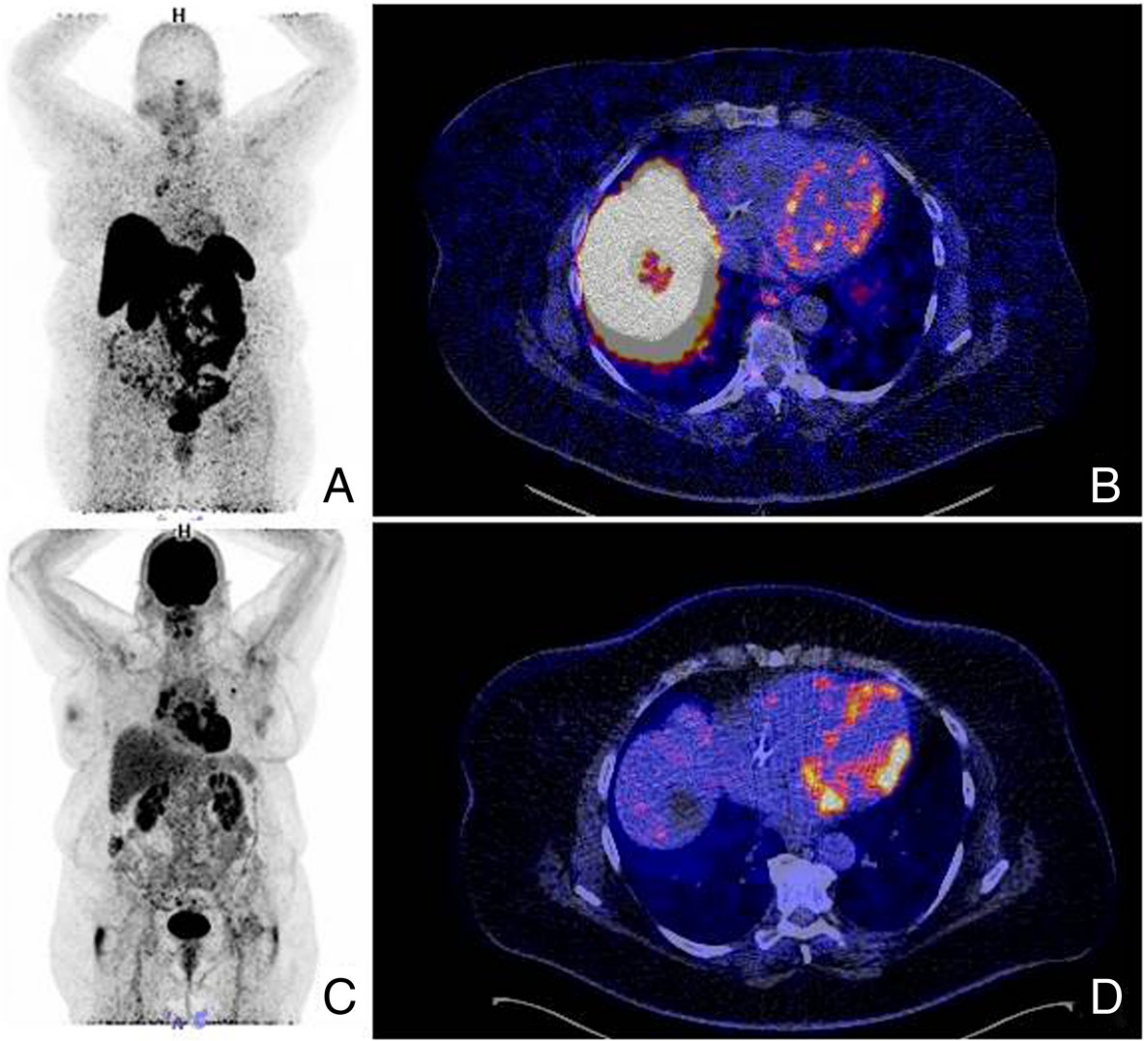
^68^Ga-Dotatoc whole body PET (a) in a 56-year old woman with chronic (cardiac) sarcoidosis. Increased uptake is visible in the lymph nodes located at the right-sideof the mediastinum and in lung hilar tissue. ^68^Ga-Dotatoc PET/CT uptake (b) is visible in the left ventricle of the heart (lateral, basal, septal) and the anterior part of the right ventricle. The same distribution pattern is visible on FDG whole body (c) and cardiac PET (d), but is more intense. Apparently FDG PET was more helpful than ^68^Ga-Dotatoc in the chronic phase of sarcoidosis in this patient
